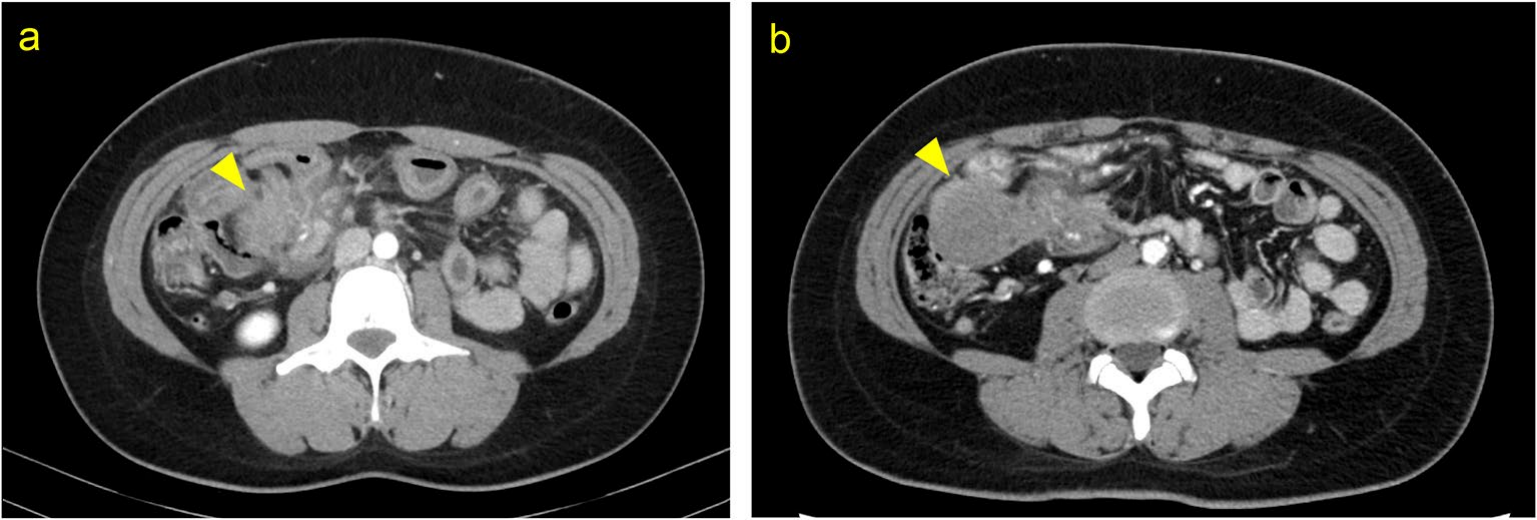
Images of contrast-enhanced computed tomography (CT). a CT images showing ileum with severe wall thickening and increase in surrounding fat concentration around the lesion at previous hospital (yellow triangle). b Follow-up CT images showing morphological changes of lumpy ileum at our hospital (yellow triangle)
